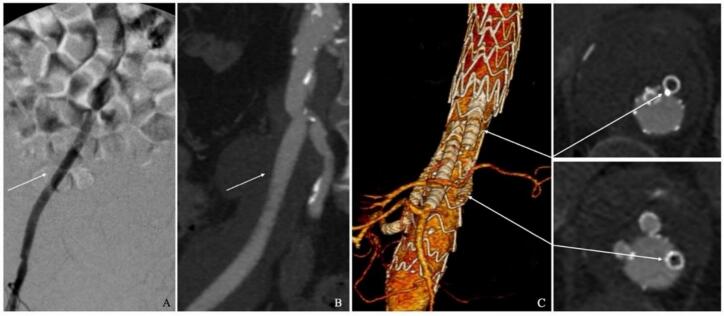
Digital Subtraction Angiography (DSA, 3 A) and post-operative Computed Tomography Angiography (CTA; 3B) revealed no extravasation of dye and excellent flow in the prosthetic iliac-to-femoral bypass (white arrows), with the patency of the internal iliac artery. C) Amplatzer Vascular Plugs I (discs' diameter of 12 mm) at the level of the inner branch of celiac trunk (CT) and a Cera Vascular Plug (discs' diameter of 10 mm) at the level of the inner branch of RRA (white arrows), without signs of endoleaks.
